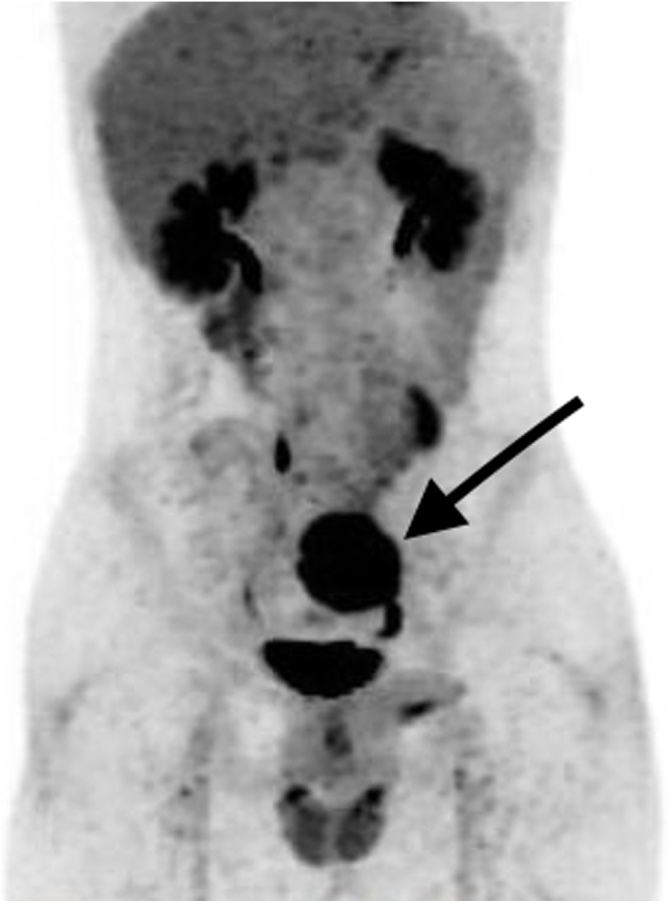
PET scan showing moderate FDG avidity within well circumscribed rounded mass in the left pelvis measures 6.2 × 5.7 cm axially.
